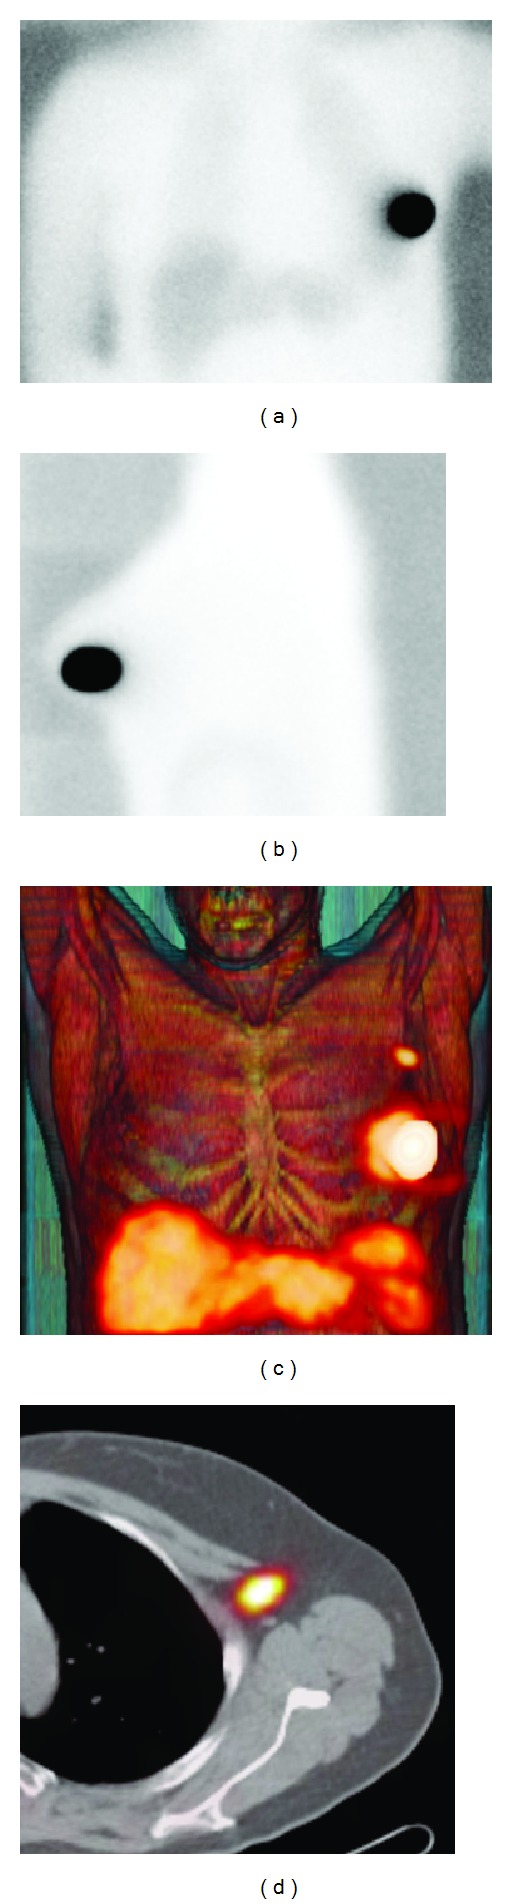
Planar images (a)-(b) showing no drainage of ^99m^Tc-nanocolloid from the injection site in left breast. By contrast, fused SPECT/CT with volume rendering (c) shows drainage to the left axilla with one sentinel node in level 1 (d).
